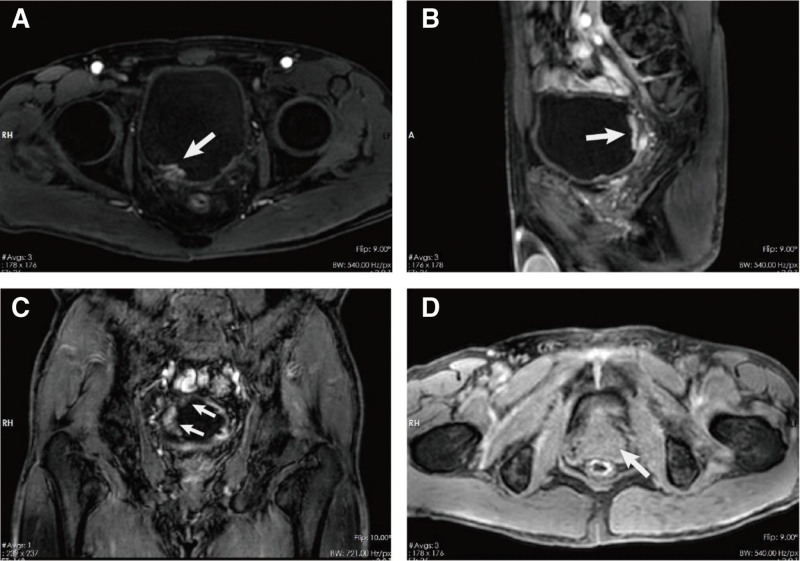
MRI of the patient before surgery. MRI showed multiple lesions on the bladder wall in the transverse (A), sagittal (B), and coronal planes (C), with the larger one located on the posterior wall of the bladder, measuring approximately 1.6 × 1.0 × 0.55 cm. (D) No abnormalities were detected in the prostate. MRI = magnetic resonance imaging.
